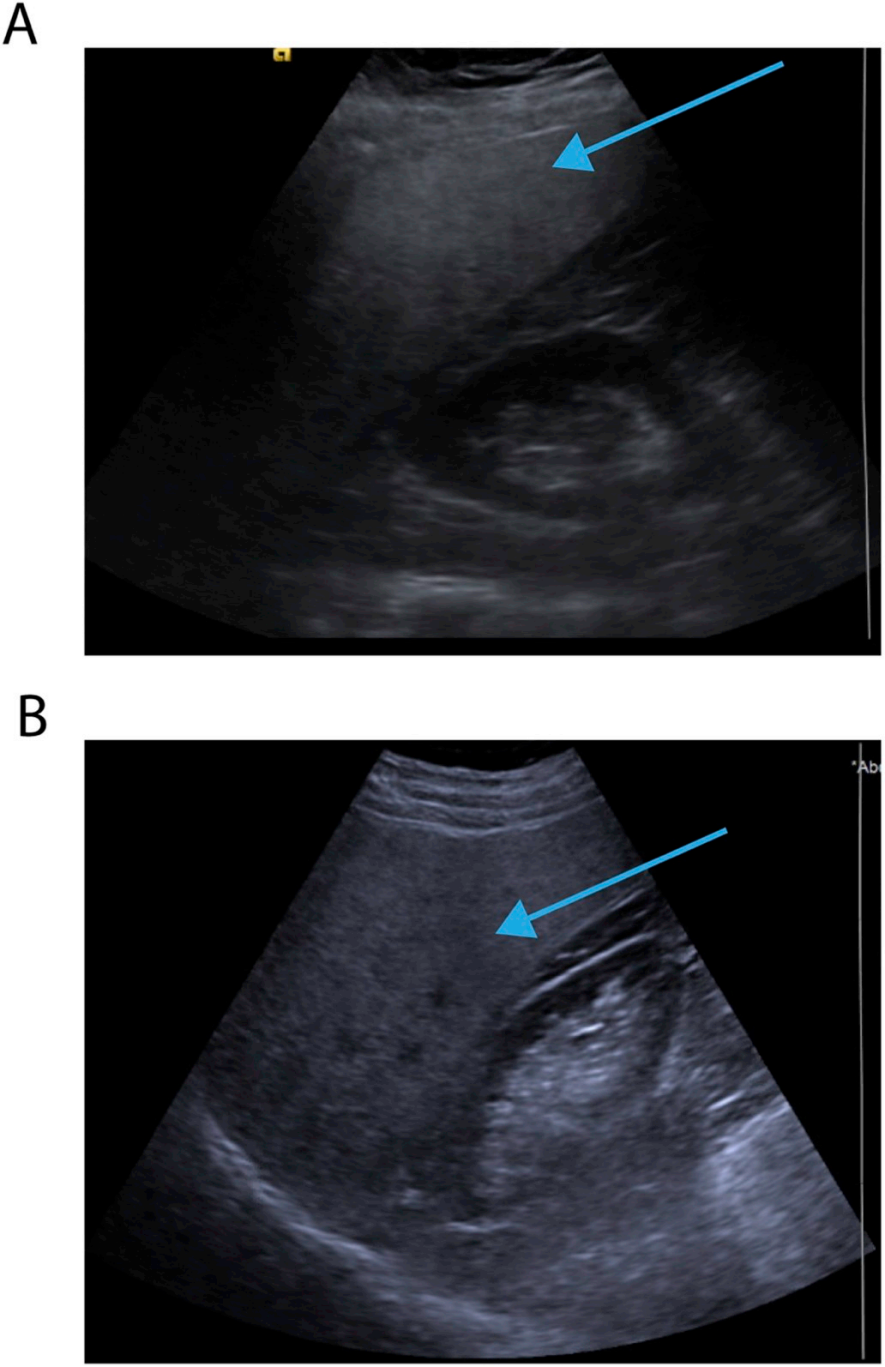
Ultrasonographic evidence of hepatic steatosis. The blue arrow highlights the increased echogenicity of the liver. (For interpretation of the references to colour in this figure legend, the reader is referred to the Web version of this article.)
